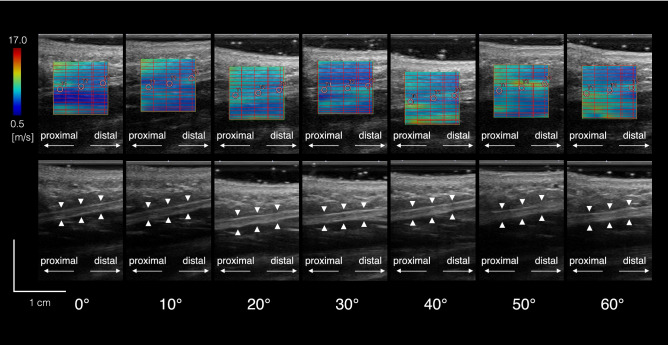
Representative ultrasound shear wave and B-mode images of the PA (upper and lower rows, respectively) that were obtained at 0°, 10°, 20°, 30°, 40°, 50°, and 60° of MP joint dorsiflexion under the weight-bearing condition. The PA is indicated by white triangles. The scale for the color code is provided to the left as SWV.
